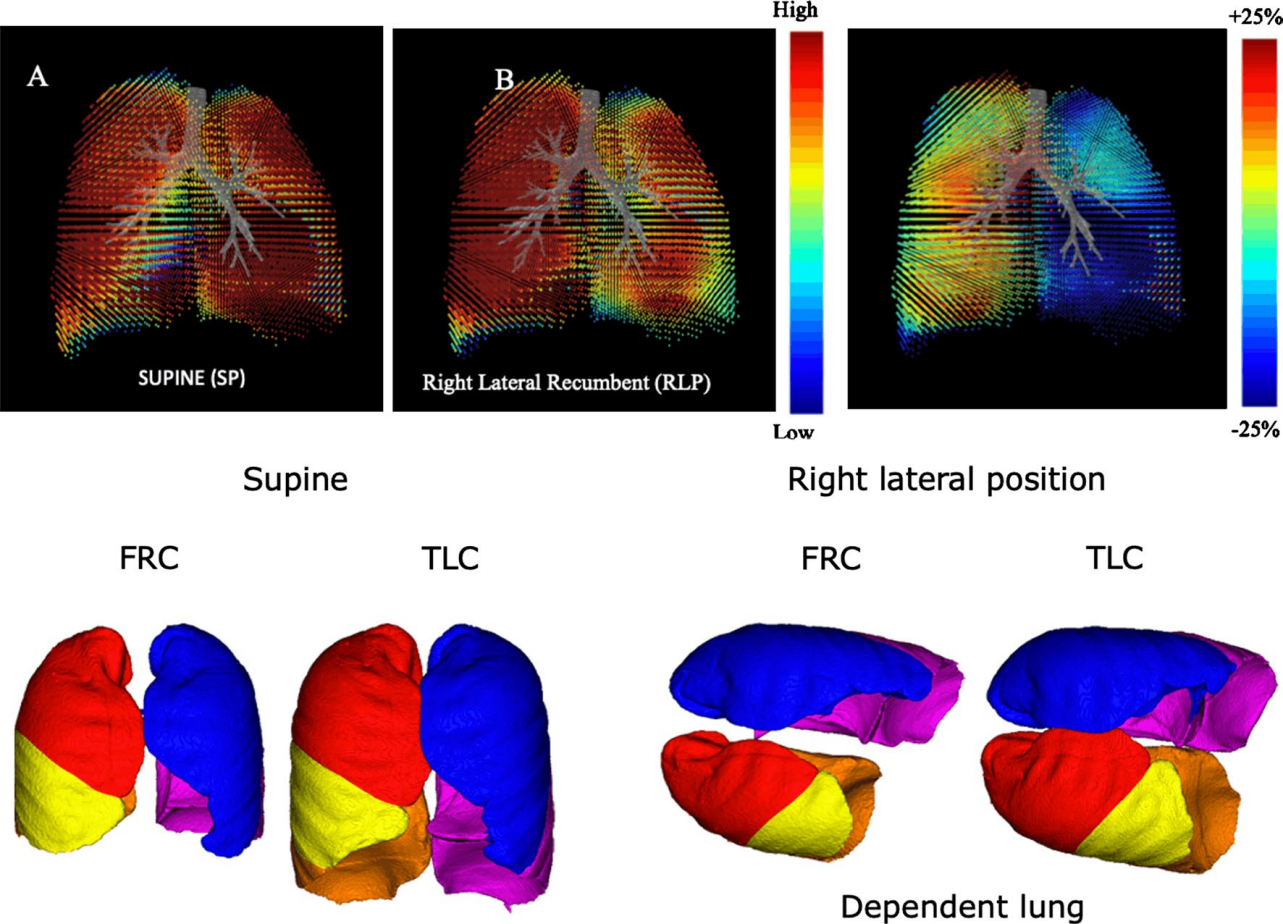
Regional ventilation maps for a healthy subject in supine (A) and right lateral position (B), as well as the relative effect between both. substantial relative ventilation defect can be seen in the left lung as a result of the right lateral position, with regional effect values up to 25. Ventilation maps are CT based representations of regional ventilation, derived from pulmonary CT images acquired at two different inflation levels, functional residual capacity (FRC) and total lung capacity (TLC) without the use of exogenous contrast, by assuming that regional changes in lung volume relate to regional ventilation The Jacobian determinant of the deformation field from FRC to TLC is calculated, which measures the differential expansion of the image
